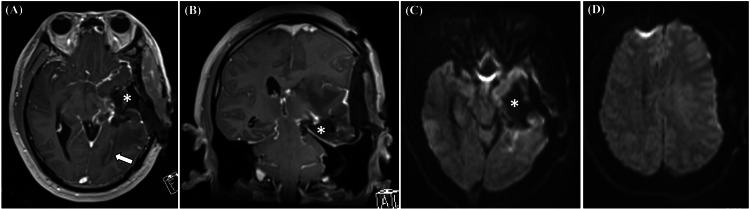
Intraoperative MRI findings Intraoperative brain MRI demonstrated gross total resection of the enhanced tumor (asterisks), except for the subependymal extension of the posterior horn of the lateral ventricle (arrow) on post-contrast T1WIs of the axial (A) and coronal (B) sequences. No obvious bleeding or ischemic complications were observed on DWI (C and D). T1WI: T1-weighted image; DWI: diffusion-weighted image; MRI: magnetic resonance imaging
